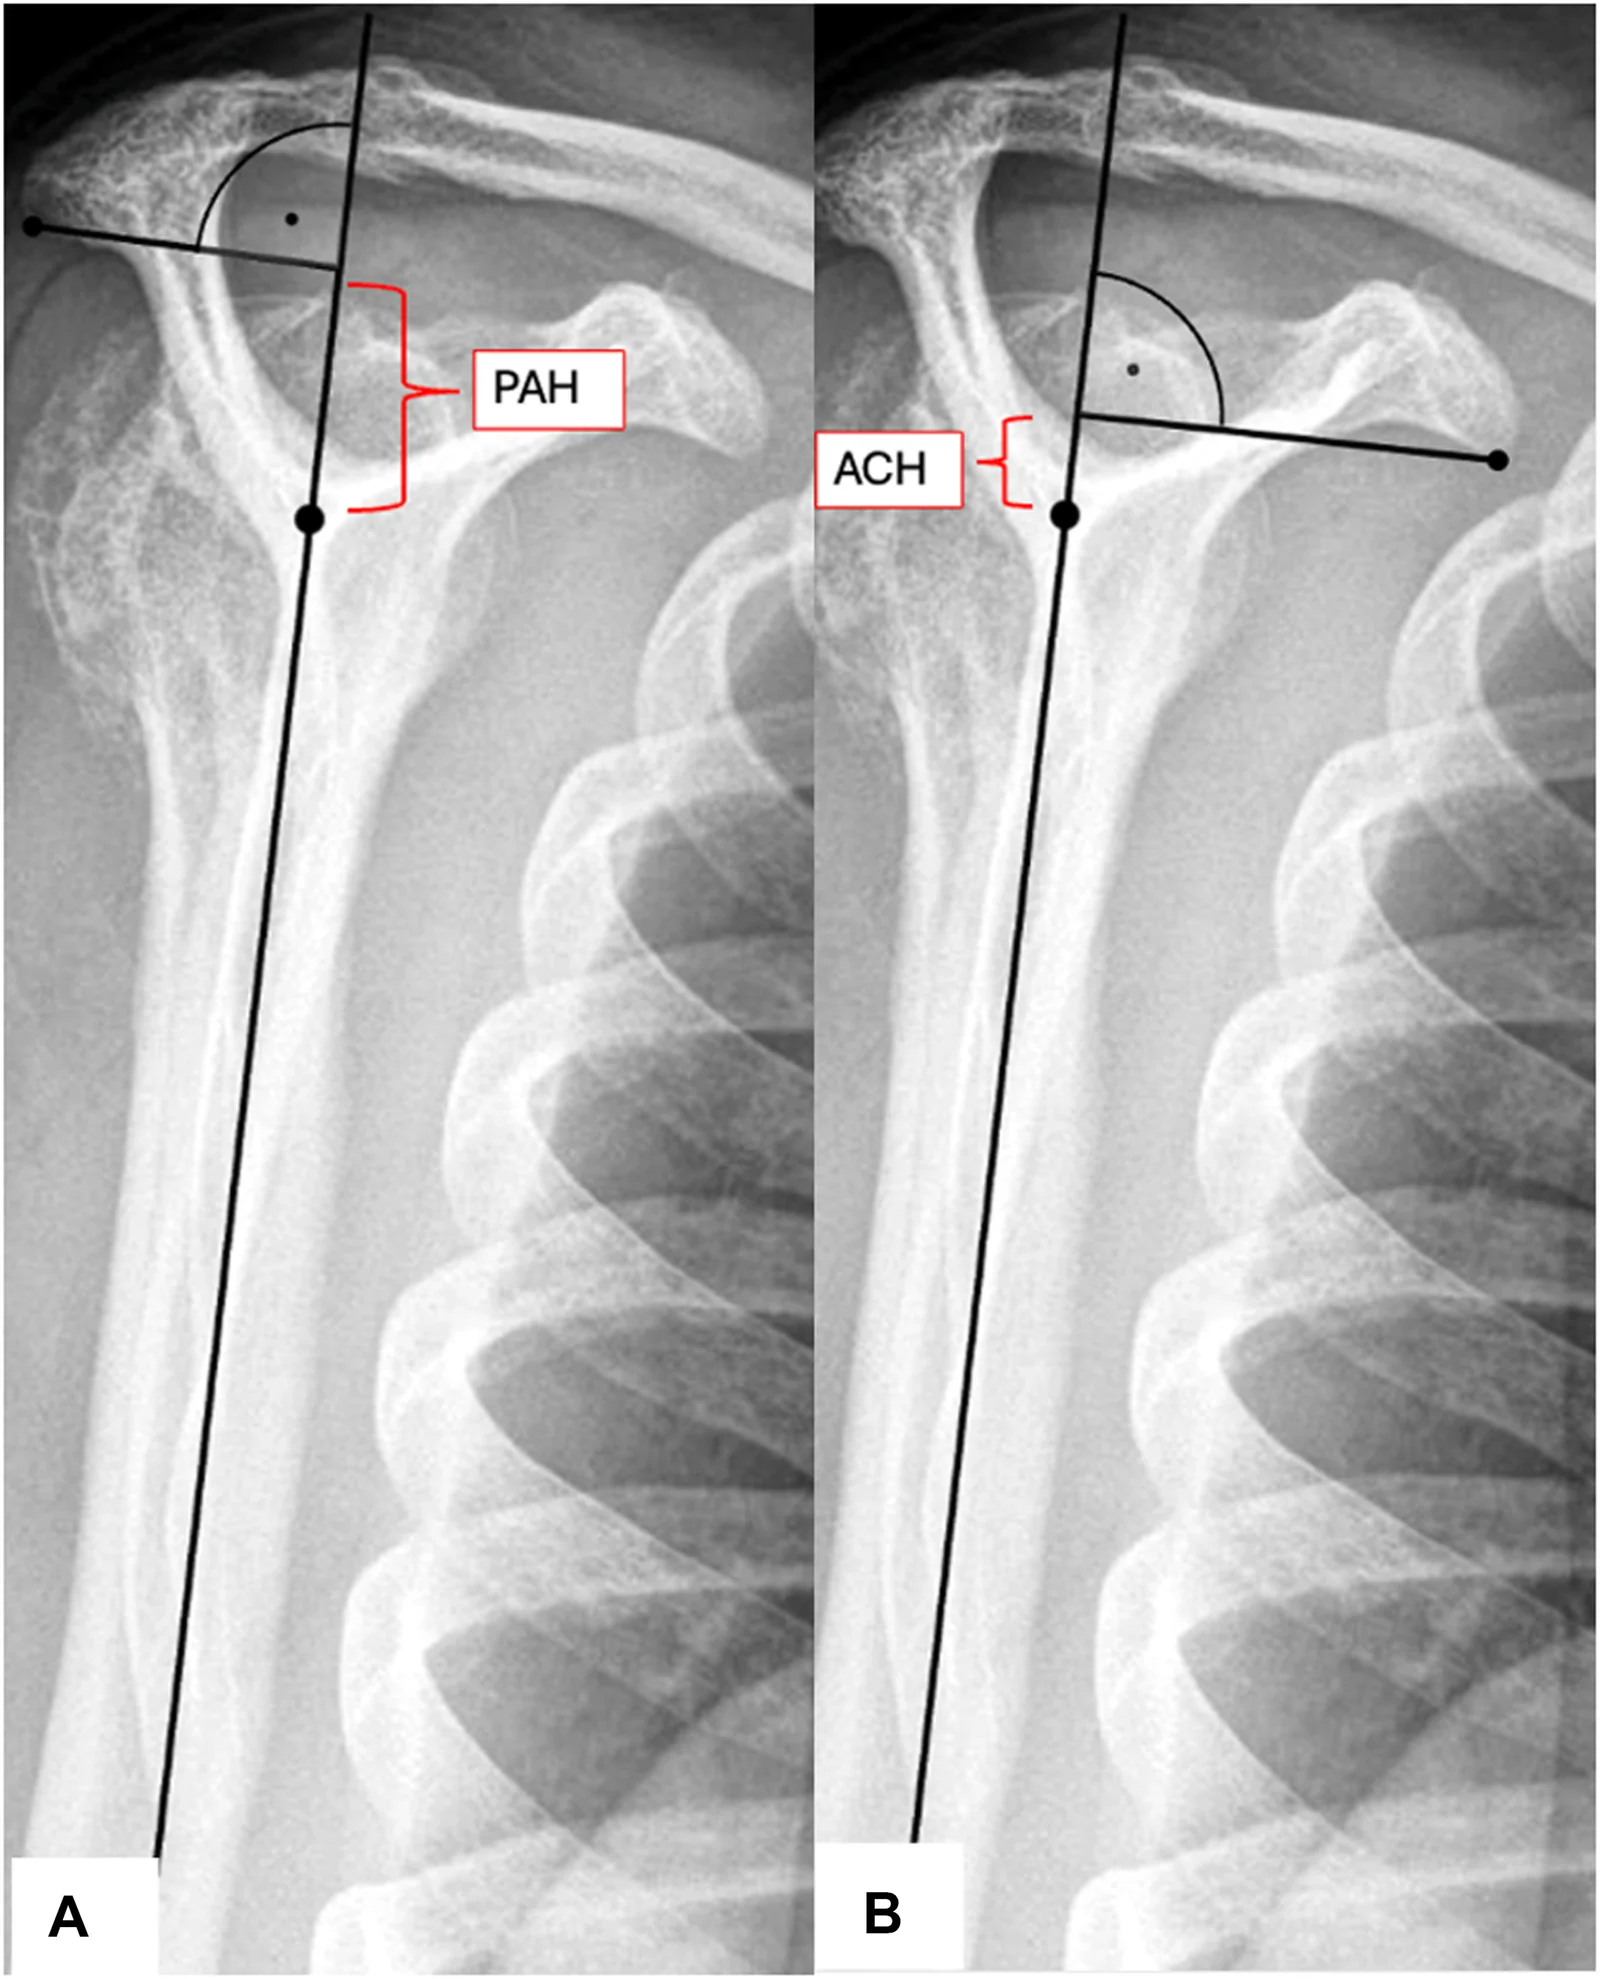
Vertical glenoid roof measurements on standardized Neer lateral Y-view radiographs. (A) Posterior acromial height (PAH) was defined as the perpendicular distance from the glenoid *Center* to the most posterior-inferior cortical point of the acromion, measured orthogonally to the vertical scapular reference axis. (B) Anterior coracoid height (ACH) was defined as the perpendicular distance from the glenoid *Center* to the most inferior cortical point of the coracoid, measured orthogonally to the same reference axis.
